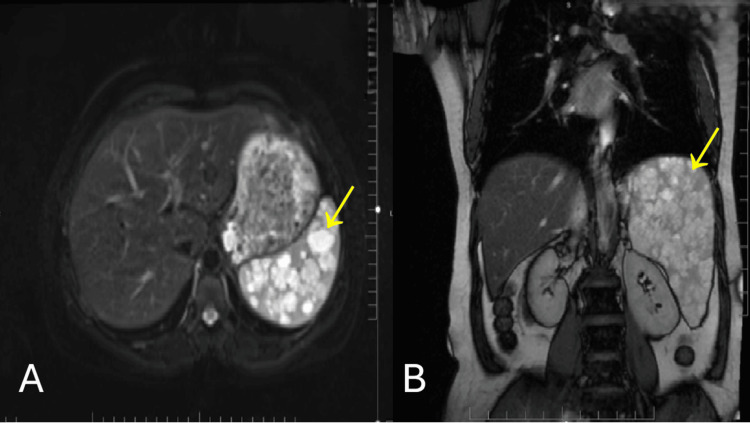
Contrast-enhanced abdominal MRI (December 8, 2021). A. Axial post‑contrast MRI demonstrating marked splenomegaly with innumerable solid and cystic lesions nearly replacing the splenic parenchyma, with heterogeneous progressive enhancement (yellow arrow). B. Coronal non‑contrast MRI from the same examination showing diffuse nodular replacement of the spleen, while the remaining abdominal organs appear preserved (yellow arrow).
